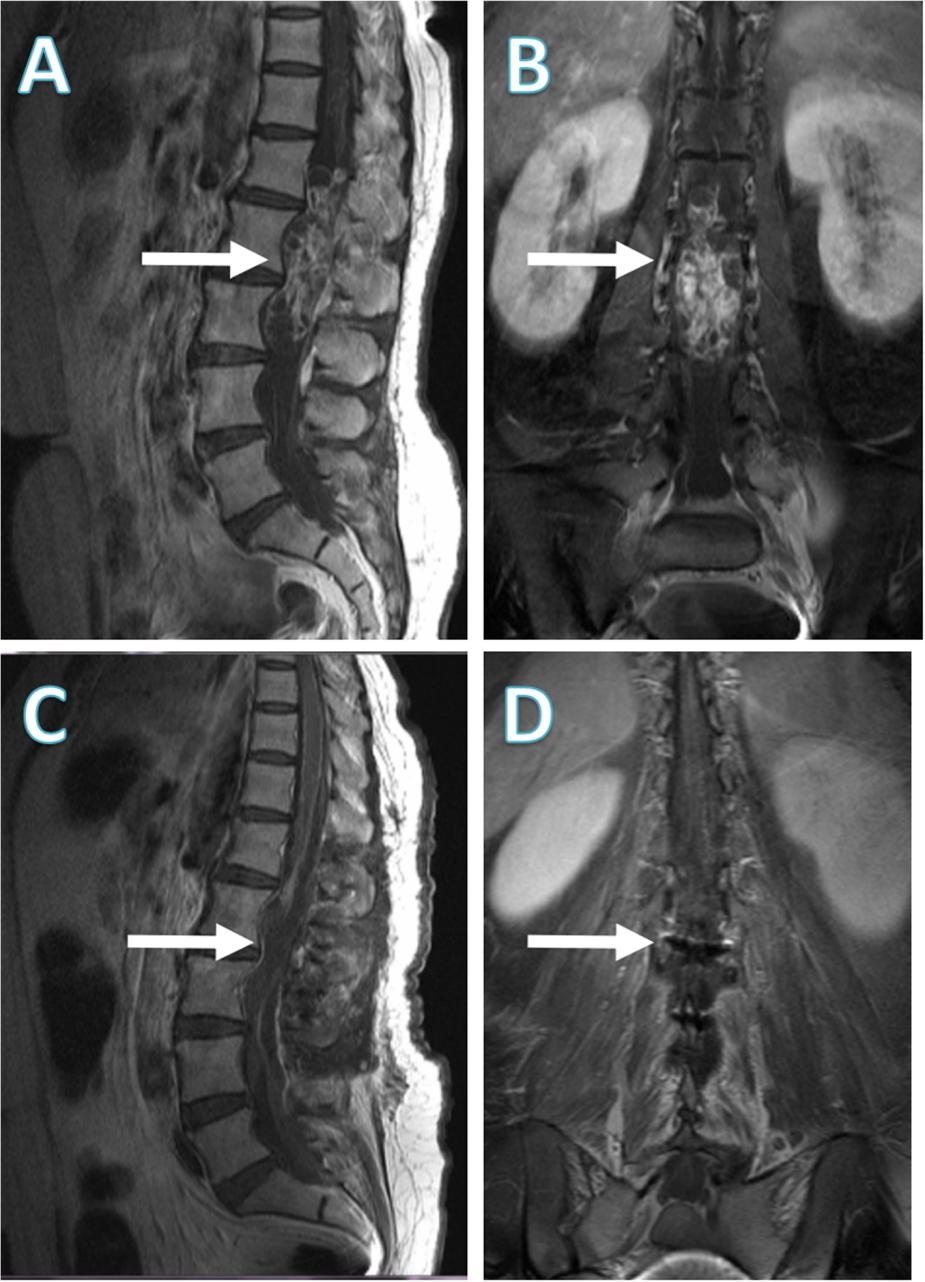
Lumber magnetic resonance imaging (MRI) examinations before and after tumor resection. a, b The preoperative lumbar MRI indicated an occupying lesion at the 1–3 lumbar vertebrae (arrow). c, d The postoperative lumbar MRI indicated a complete resection of the tumor (arrow)
